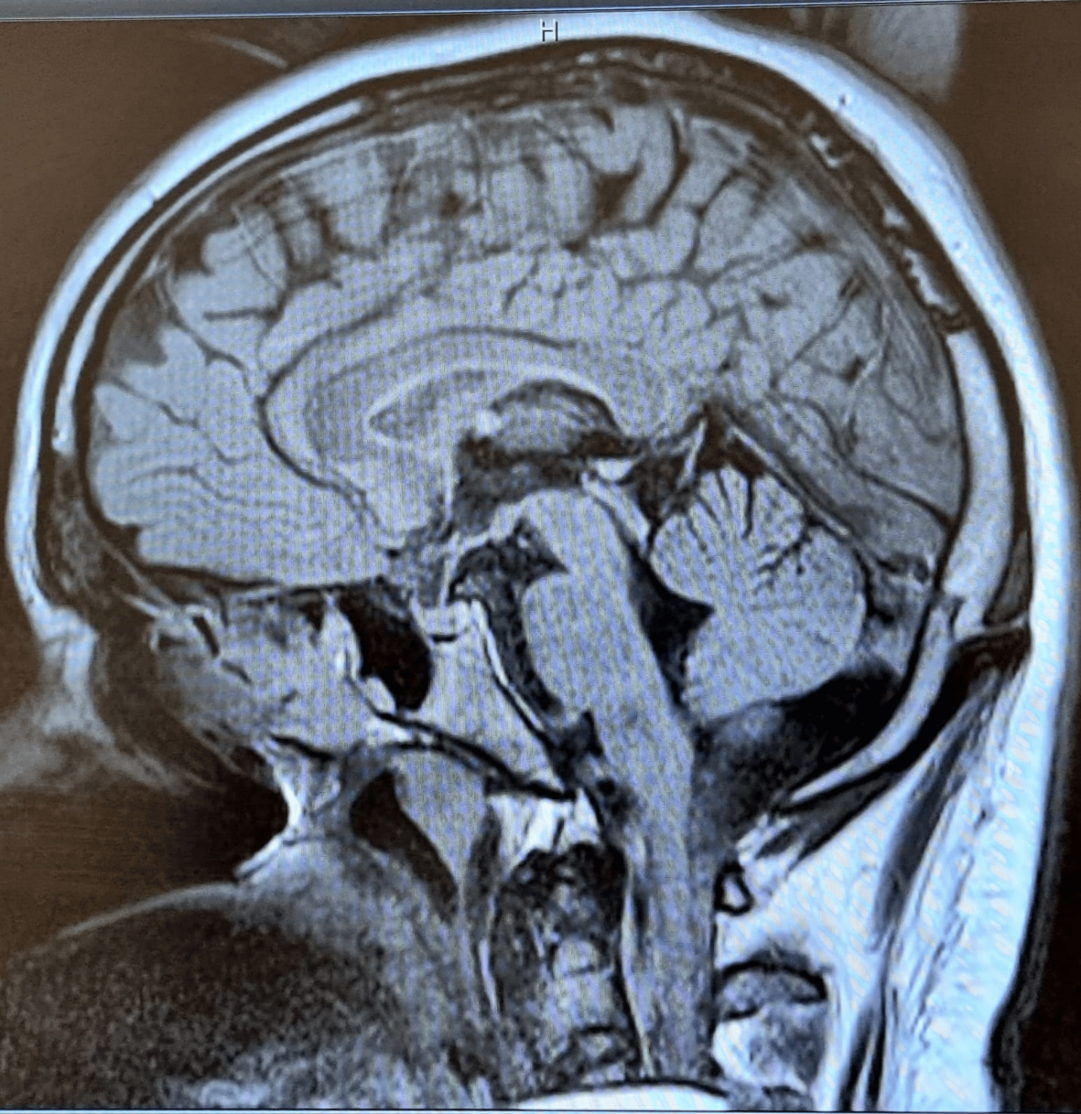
The MRI of Case 1's brain (sagittal T1 image) shows no gross pathology.
